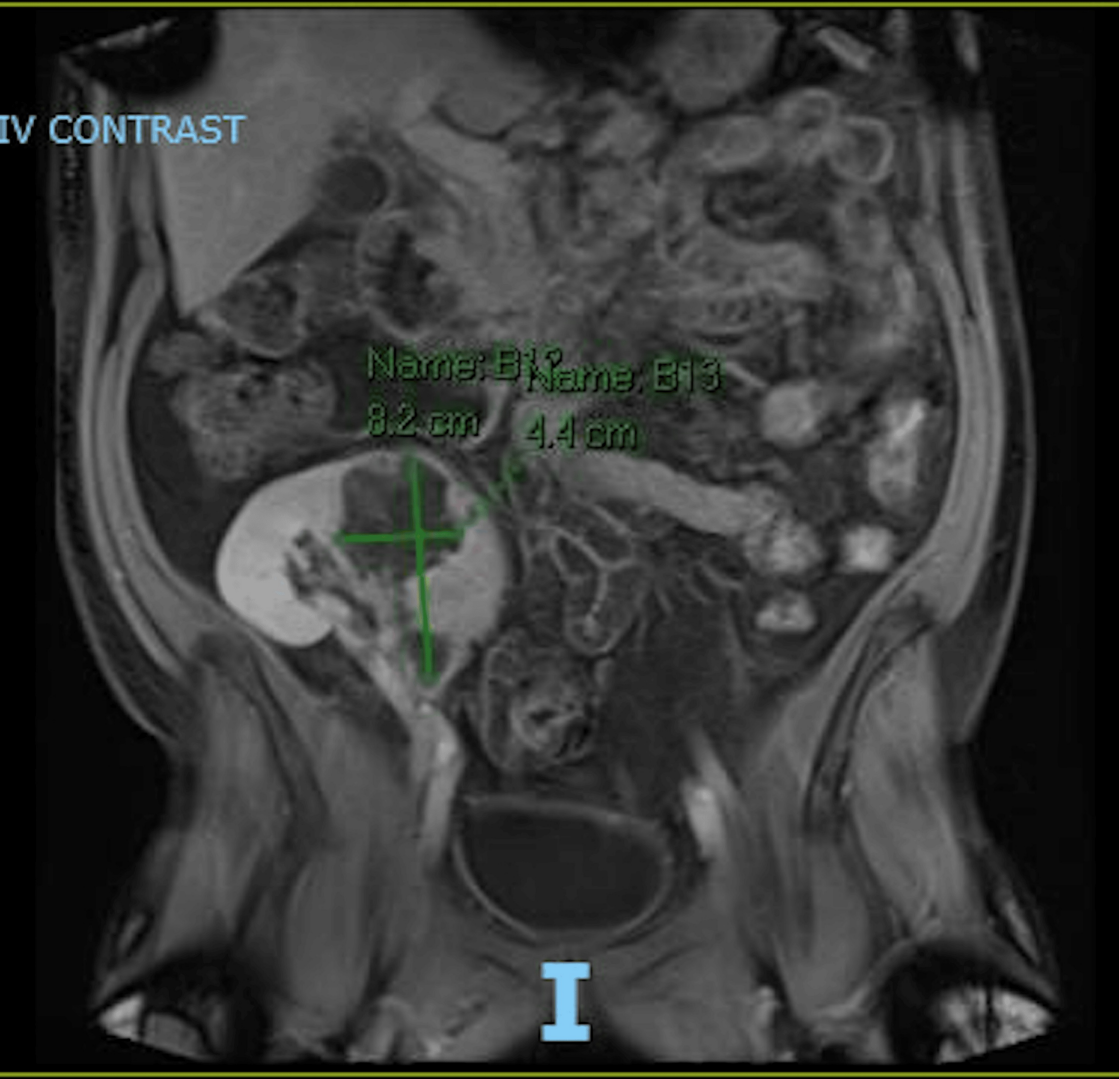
Abdominal MRI The figure depicts a non-contrast MRI showing the 4.4cm x 8.2 cm mass in the transplanted kidney.
